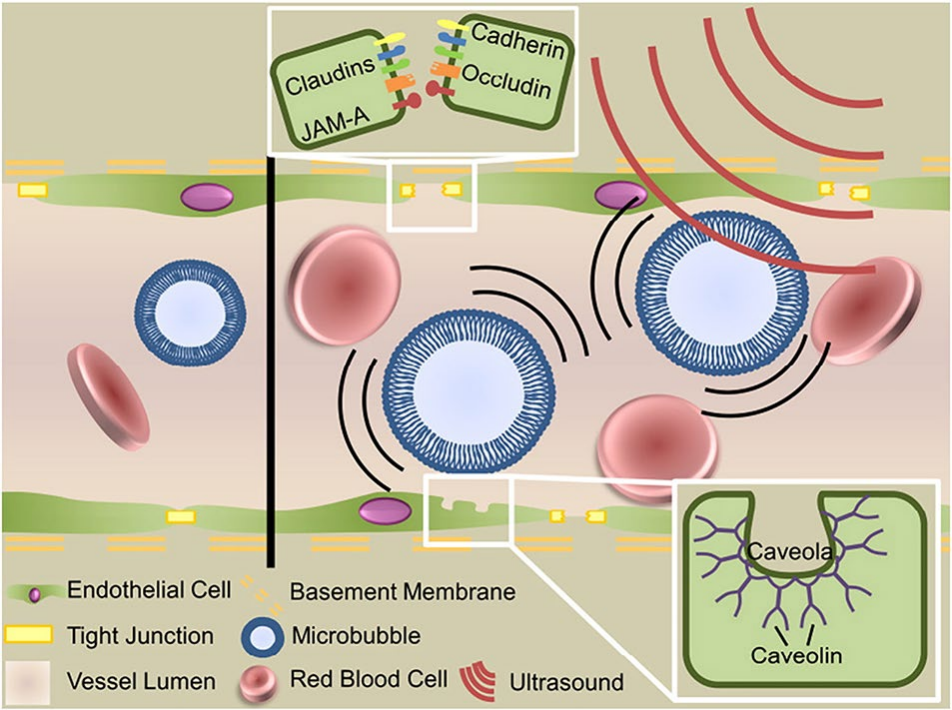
Mechanisms of focused ultrasound mediated blood–brain barrier disruption. Circulating microbubbles oscillate in the ultrasonic field, producing forces that act on the vessel wall to generate three bioeffects that permit transport across the blood–brain barrier: disruption of tight junctions, sonoporation of the vascular endothelial cells and upregulation of transcytosis. Adapted from Timbie et al. (2015). Reproduced with permission.
